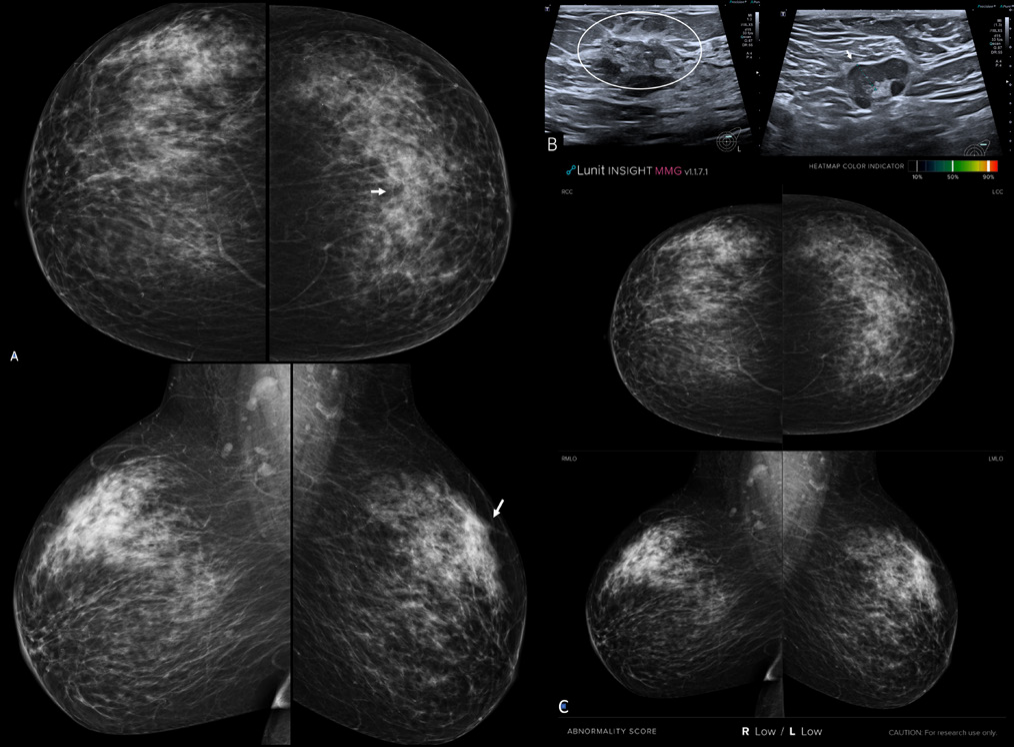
Left invasive lobular carcinoma in heterogeneously dense breasts (ACR c). A: bilateral mammograms showed indirect sign of malignancy presented by tissue peaking (arrow) seen at the left breast upper central region. B, Ultrasound image that targeted the left breast abnormality showed indistinct isoechoic solid mass and in plane with the breast tissue (BI-RADS 4). C, AI- scanned mammograms showed “Low” scoring not matching with the mammogram and the ultrasound findings. The left breast was a false negative count by AI.
